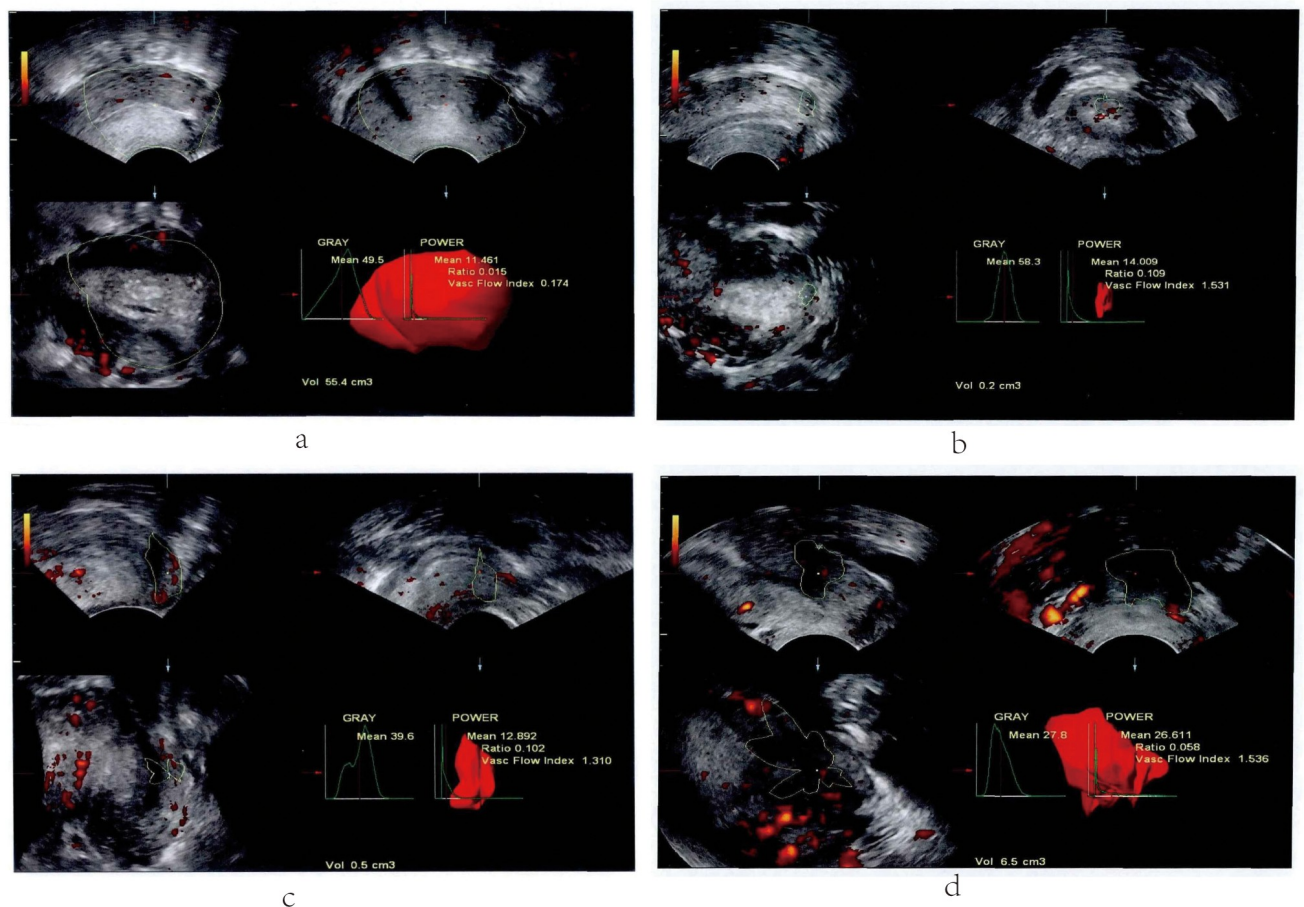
The Doppler ultrasound images on cervical cancer with different Adler grade. a, Adler 0; b, Adler I; c, Adler II; d, Adler III.
